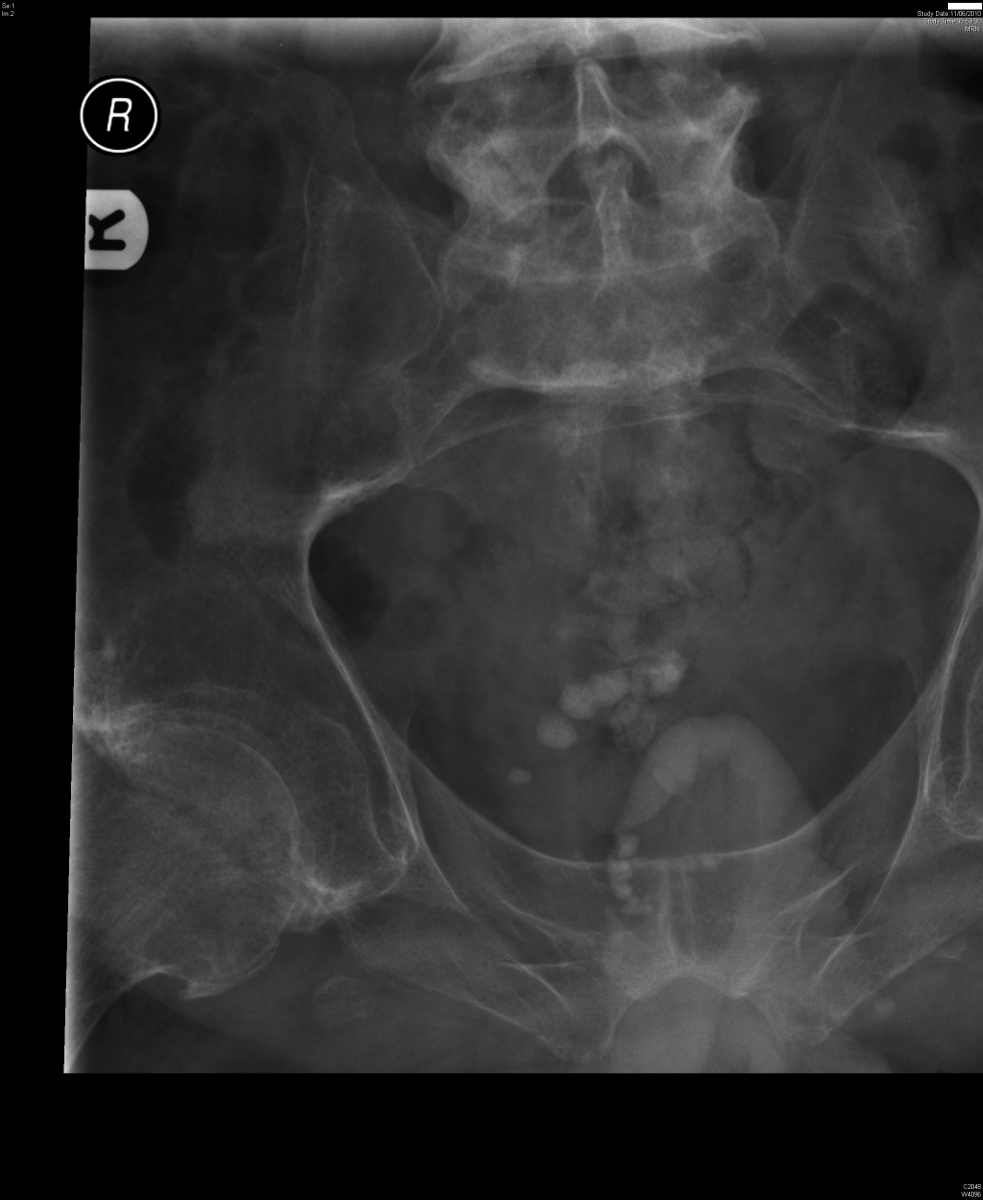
Case number: 2. X-ray of urinary bladder, taken on 11 June 2010, showed multiple calculi in urinary bladder. This patient did not develop autonomic dysreflexia when lithotripsy was performed under subarachnoid block.
